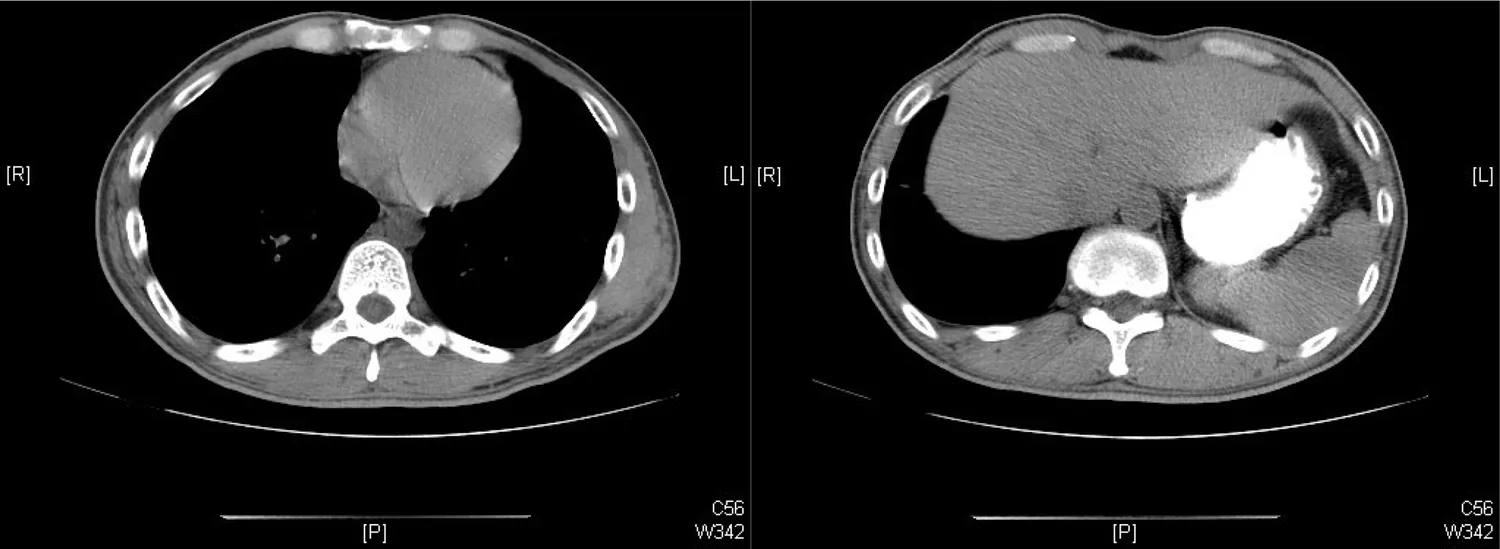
Preoperative chest CT prior to Stage II surgery showing resolution of pleural effusion and pneumomediastinum, disappearance of the oesophageal shadow, and satisfactory gastric distension.
